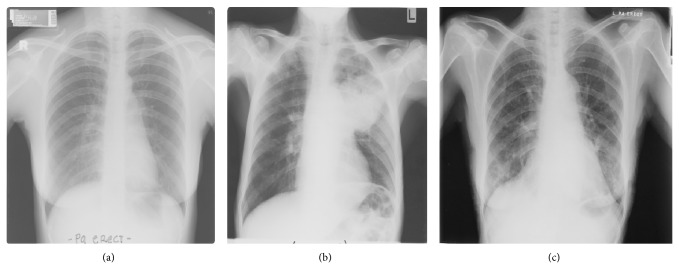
Digital chest X-rays of (a) normal lung, (b) LC in left upper zone, and (c) PNEU in right and left lower zone (source: Insitute of Respiratory Medicine, Kuala Lumpur).
